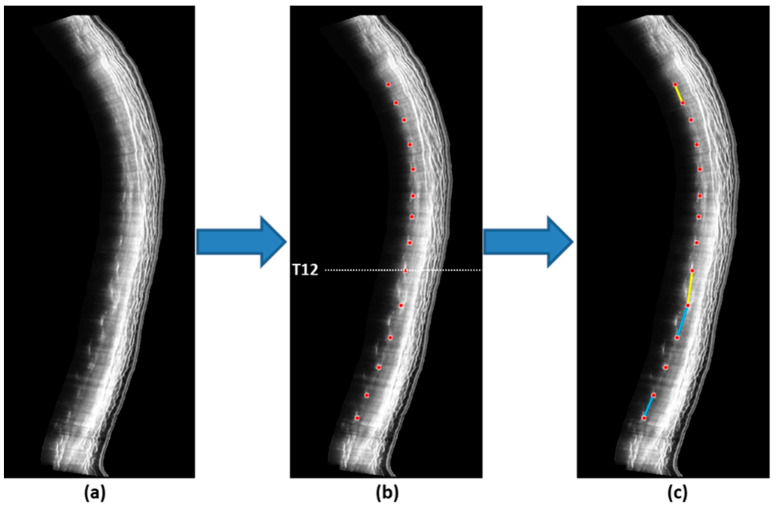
(a) Ultrasound sagittal images of the spine; (b) Locations of the laminae (red dots) were identified and the corresponding coordinates were extracted for computation of the sagittal curvatures (T12 level is indicated by the white dotted line); (c) Thoracic kyphosis was defined as the angle formed between the line T4 and T5 coordinates and the line joining T11 and T12 laminae (yellow); in contrast, lumbar lordosis was defined as the angle formed between the line L1 and L2 coordinates and the line joining L4 and L5 laminae (light blue).
